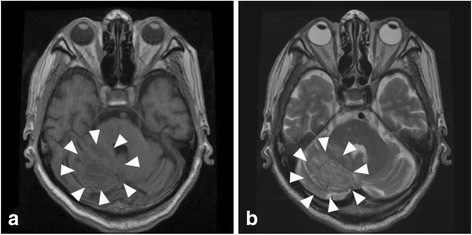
MRI findings in cerebellar dysplastic gangliocytoma. T1 (a) and T2 (b) weighted magnetic resonance images of the head. A striped pattern is seen in the right hemisphere of the cerebellum (arrowheads). The cerebral aqueduct is compressed
